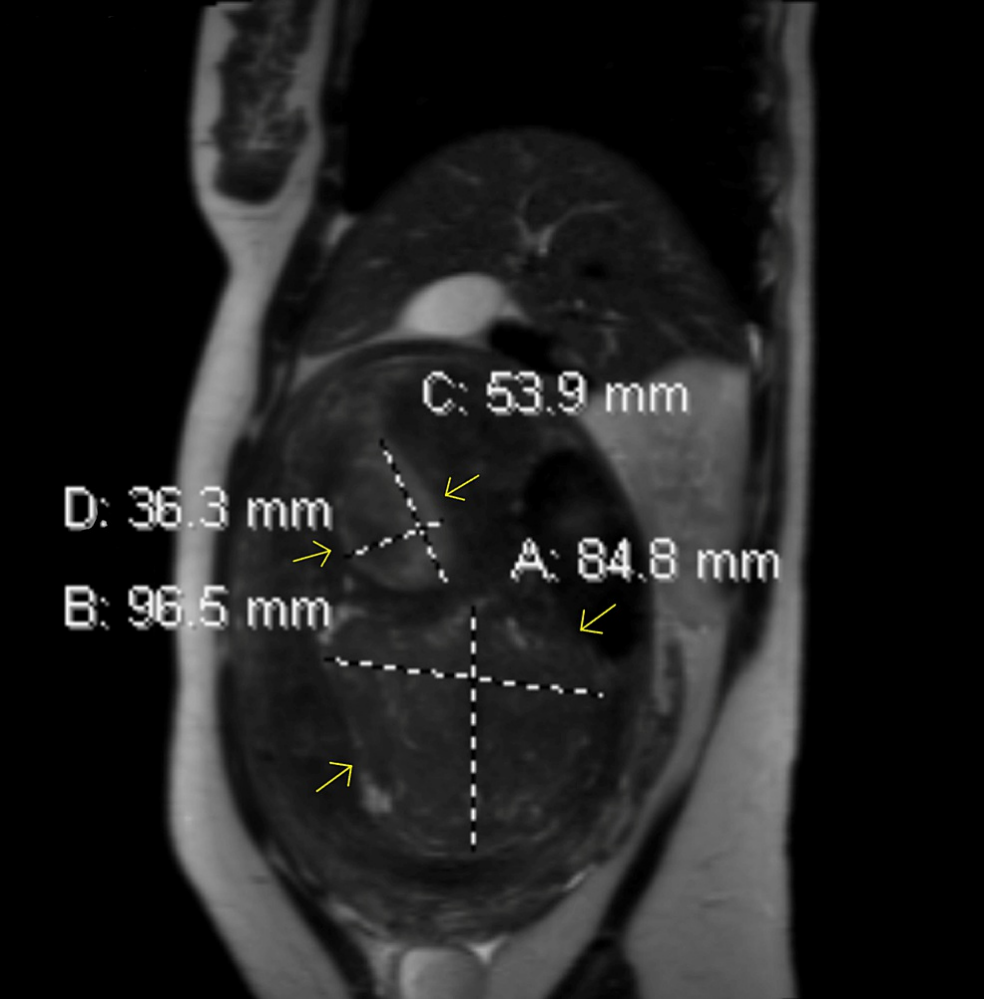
Magnetic resonance imaging displaying a sagittal view of the abdomen revealing multiple intramural fibroids (depicted by the yellow arrows) and their measurements in millimeters
